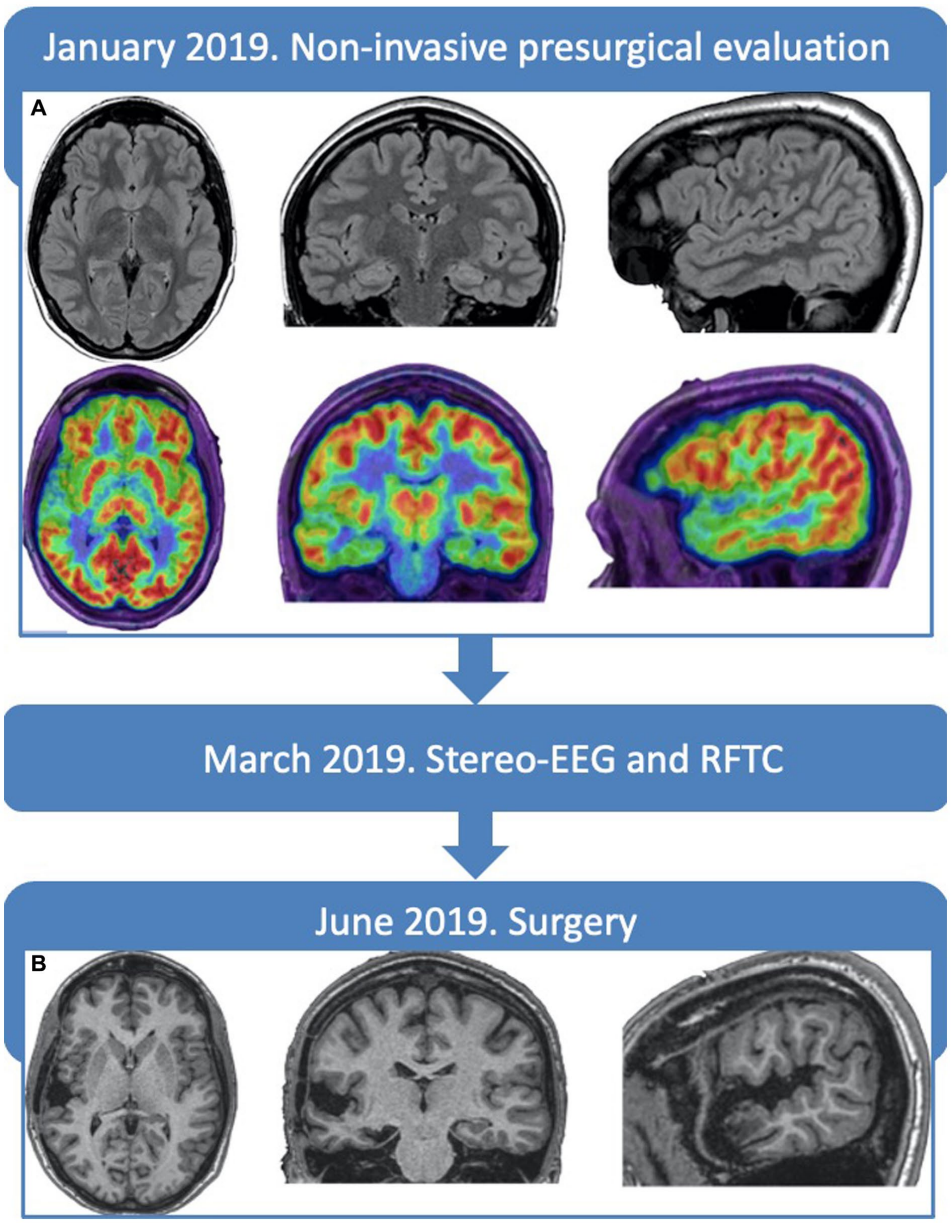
Timeline and neuroimaging. (A) Preoperative brain-MRI FLAIR slices, displaying no alterations and PET showing an hypometabolism of the right temporal lobe, more marked in the superior temporal gyrus and temporal pole. (B) Post-operative brain-MRI T13D slices, at 6 months, showing the resection cavity, without complications.
